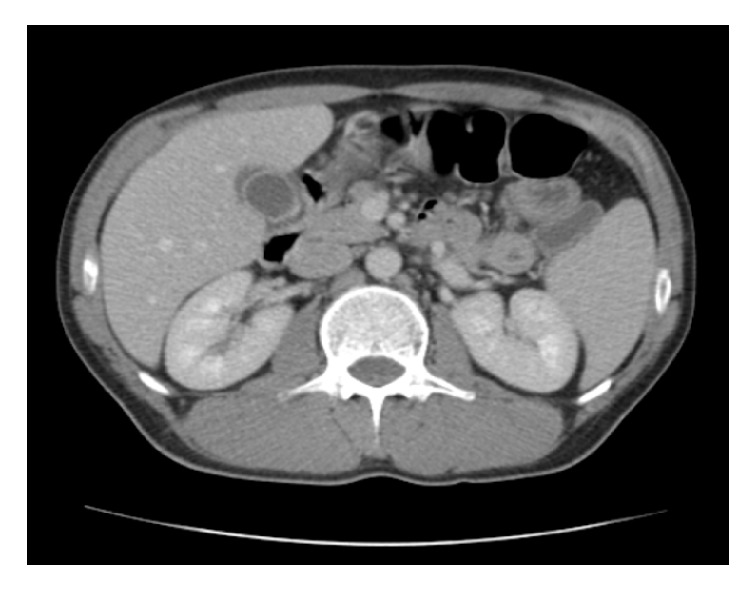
CT-scan of the abdomen showing thickening of the gallbladder wall and pericholecystic fluid collection.
